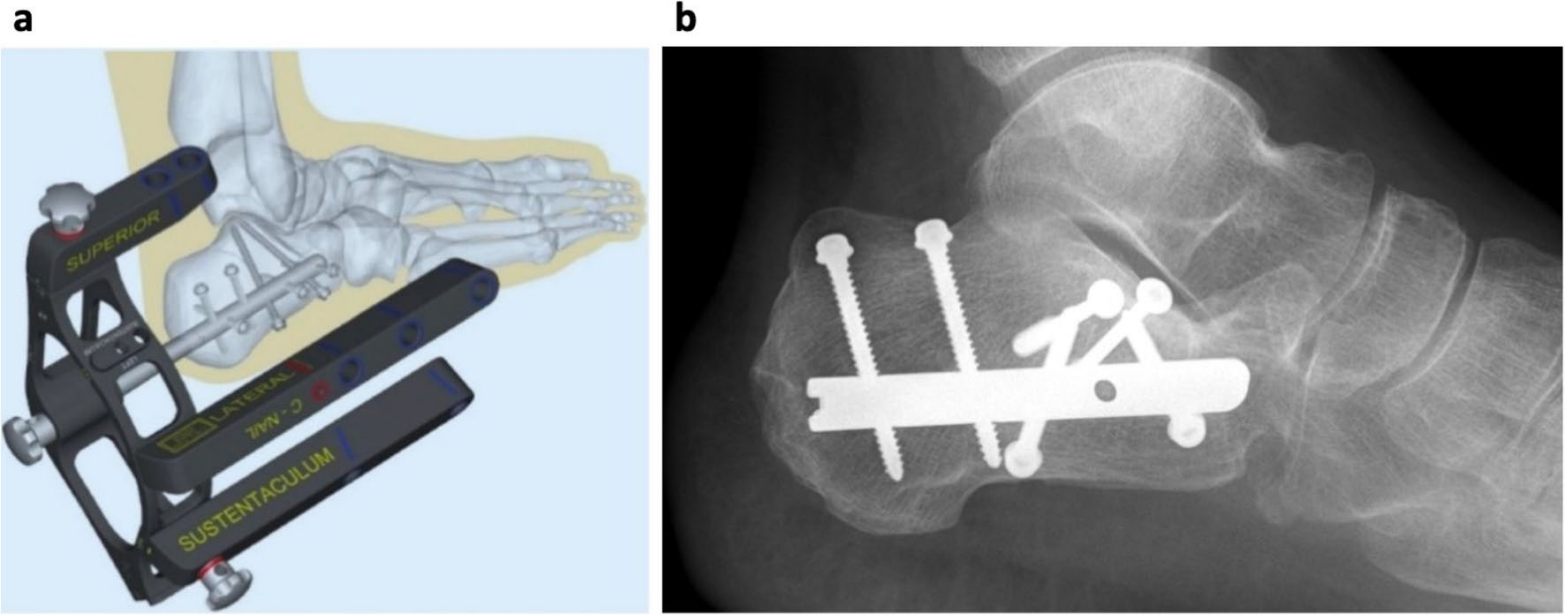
C-Nail® target instrument and exemplary postoperative X-ray*. a The sketch of the C-Nail® implant with the target instrument. Image with kind permission from Medin (Nov. Město n. Moravě, Czech Republic). b An exemplary postoperative X-ray. Here, two screws were placed through a sinus tarsi approach and four locking screws were placed through the nail. A drain was inserted at the sinus tarsi incision
